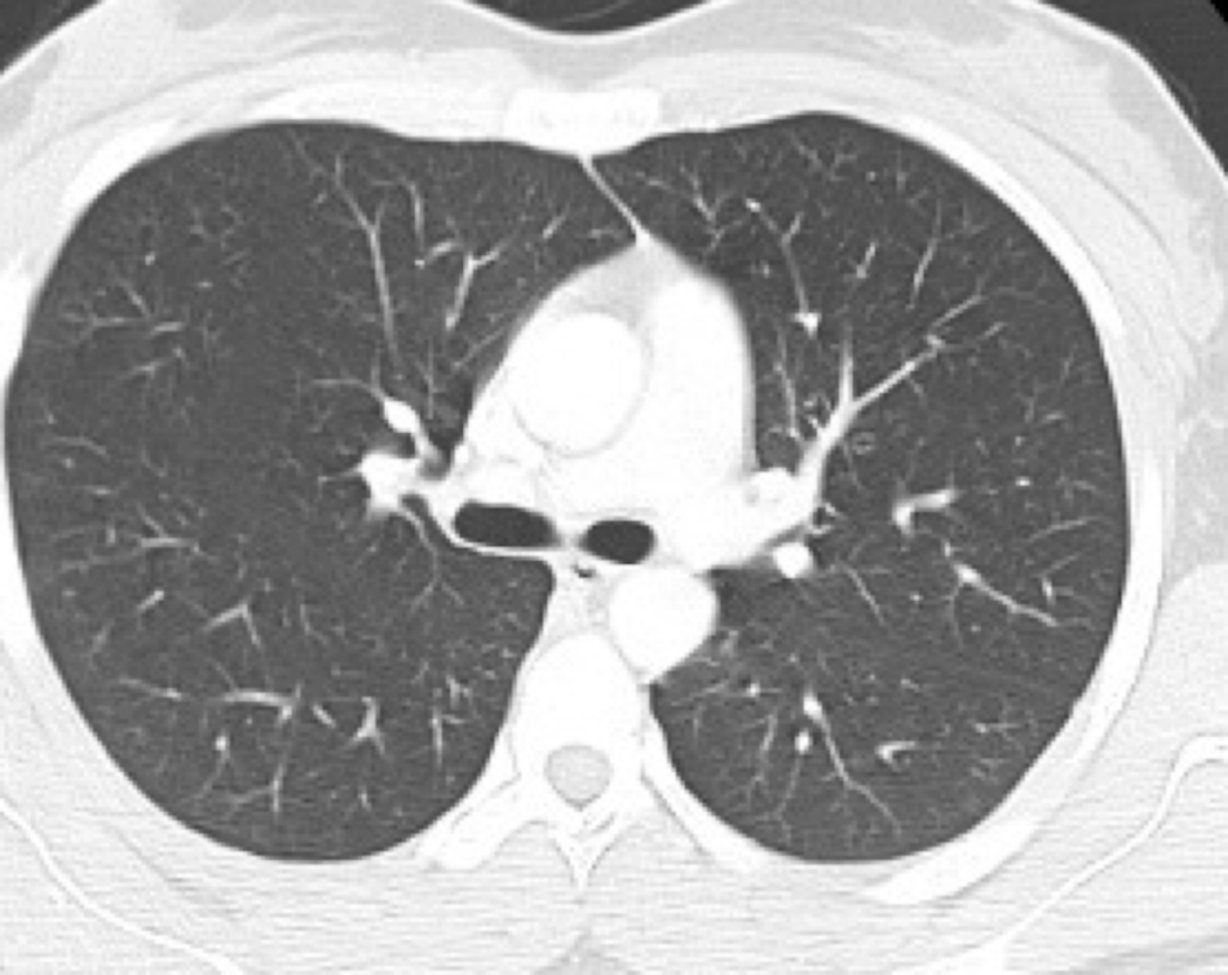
Computed tomography (CT) of the chest did not reveal any abnormality
